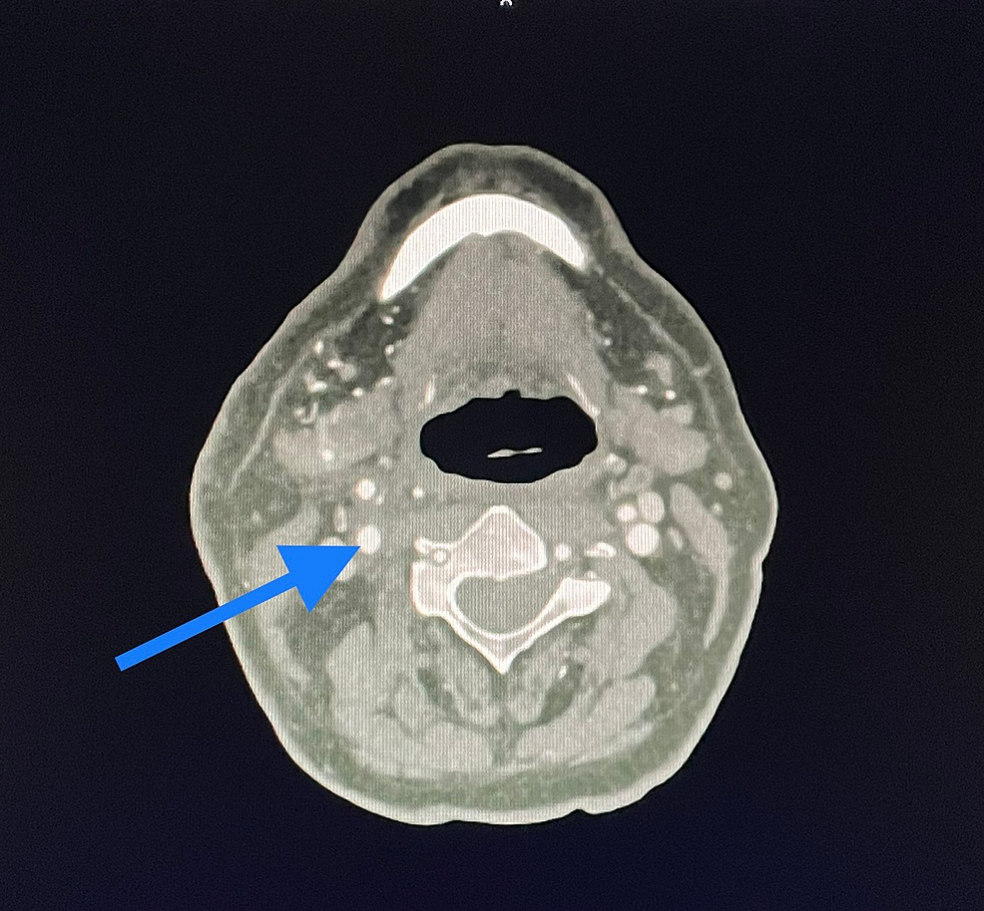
Control CT image shows no residual thrombus in right carotid arteries.
